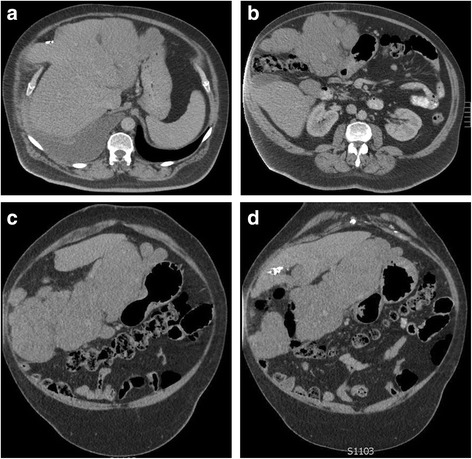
CT abdomen of recurrent disease adjacent to resection site. a–b Axial views. c–d Transverse views
